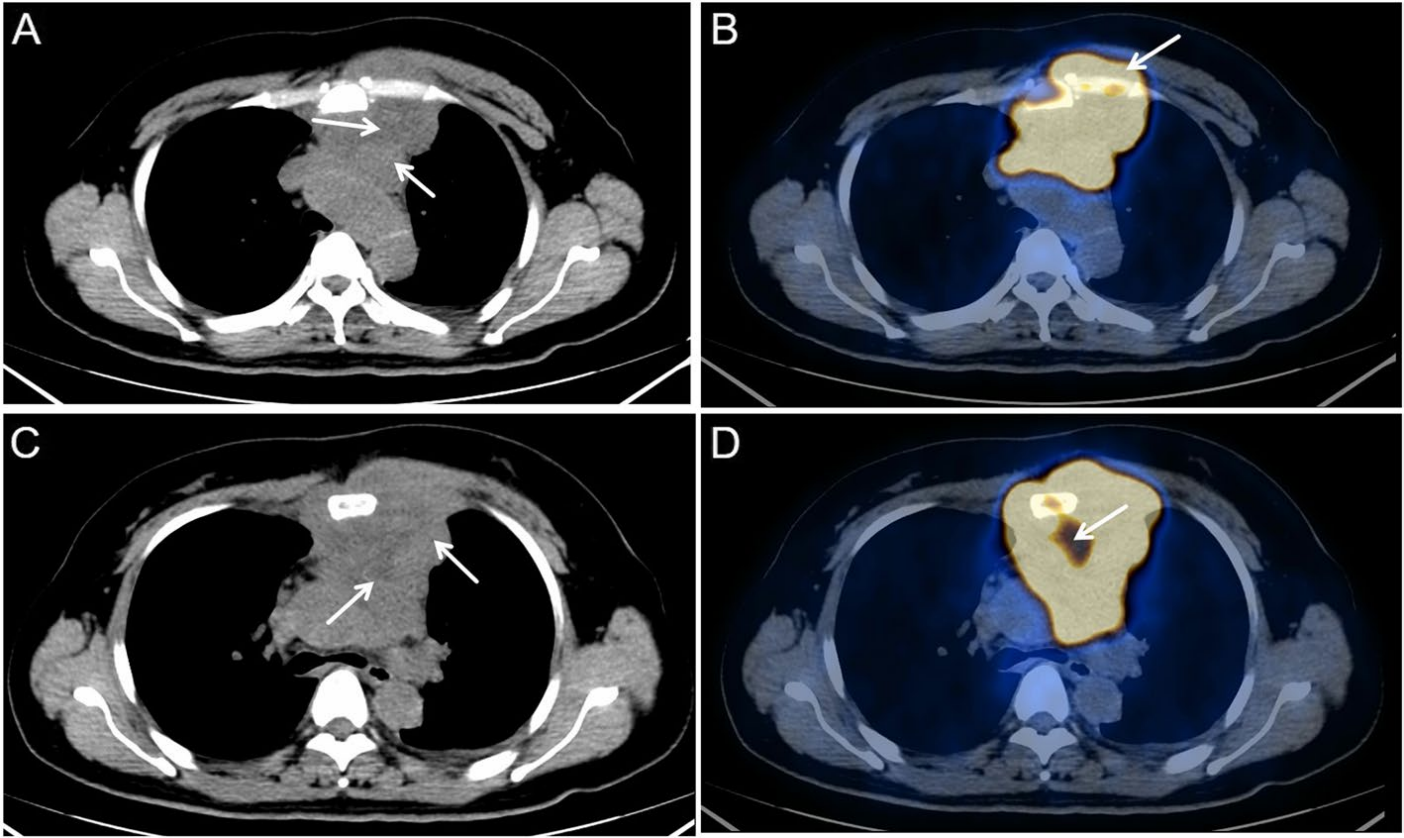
PET/CT findings of a 23-year-old patient with diffuse large B-cell lymphoma. A A large soft tissue mass in the anterior medial malleolus which was composed of multiple nodules. Tumor invaded into the front chest wall and partial adjacent large blood vessels (arrow). B Significant radioactive abnormal signals (arrow) in the tumor, SUVmax $$=$$ 35.5. C Tumor edge was lobulated with a fissure-like necrotic region inside the tumor (arrow). D Radioactive defect inside necrotic region (arrow)
